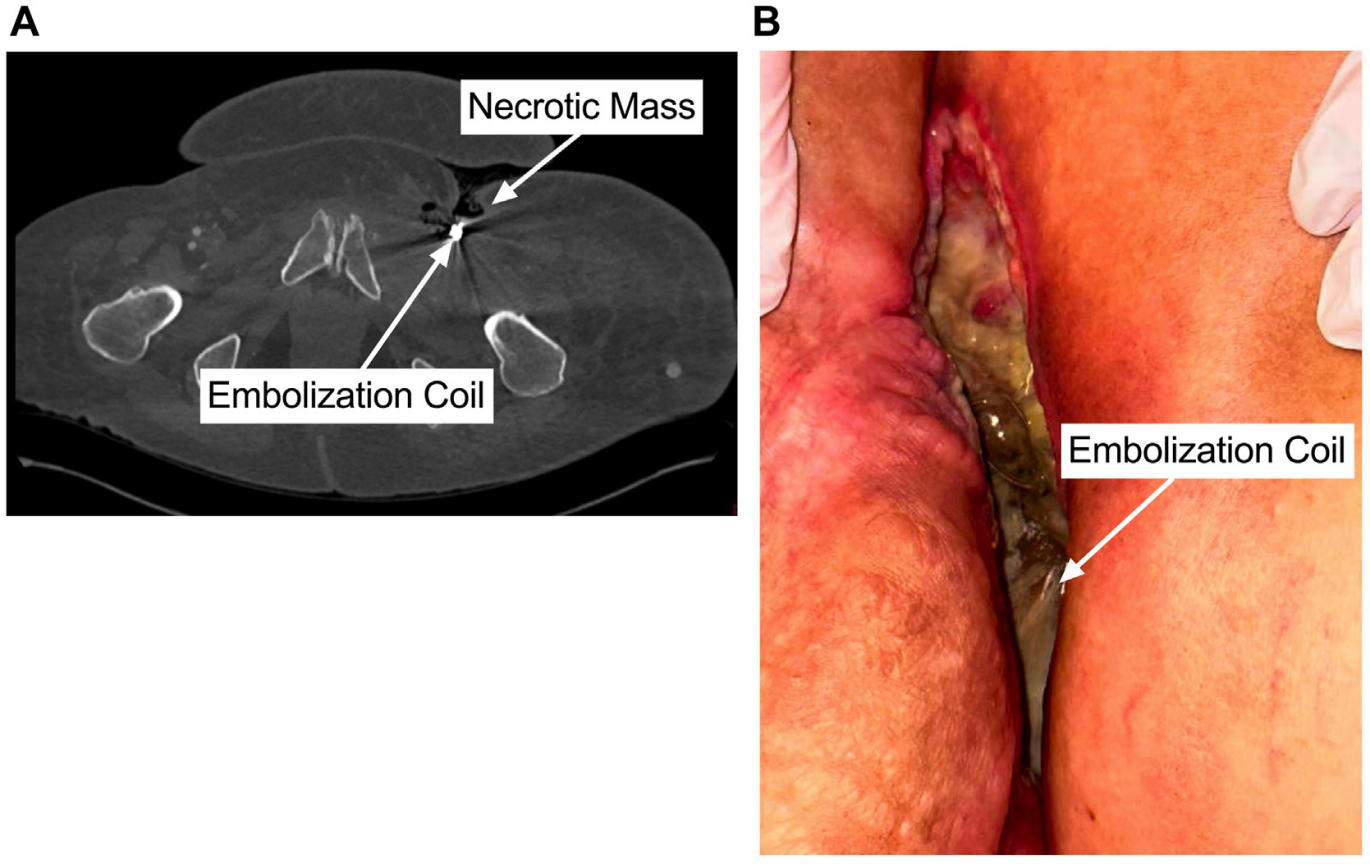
Postoperative left groin embolization coil erosion. (A) Three-month postoperative computed tomography (CT) angiography showing erosion of the embolization coil at the level of necrotic mass. (B) Three-month postoperative left groin with embolization coils eroded through the femoral artery without hemorrhage.
